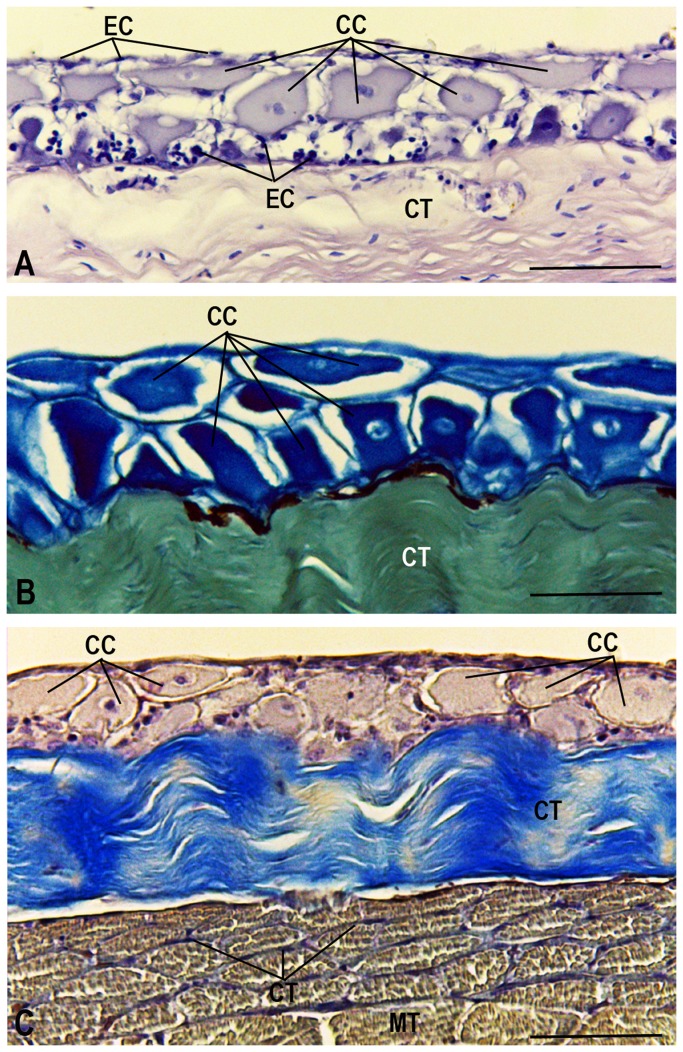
Cytochemistry. (A) PAS method; (B) Bromophenol Blue method and (C) Mallory trichrome method. Epidermal cells (EC), club cells (CC), connective tissue (CT) and skeletal muscle tissue (MT). Scale bars: 15 µm.
